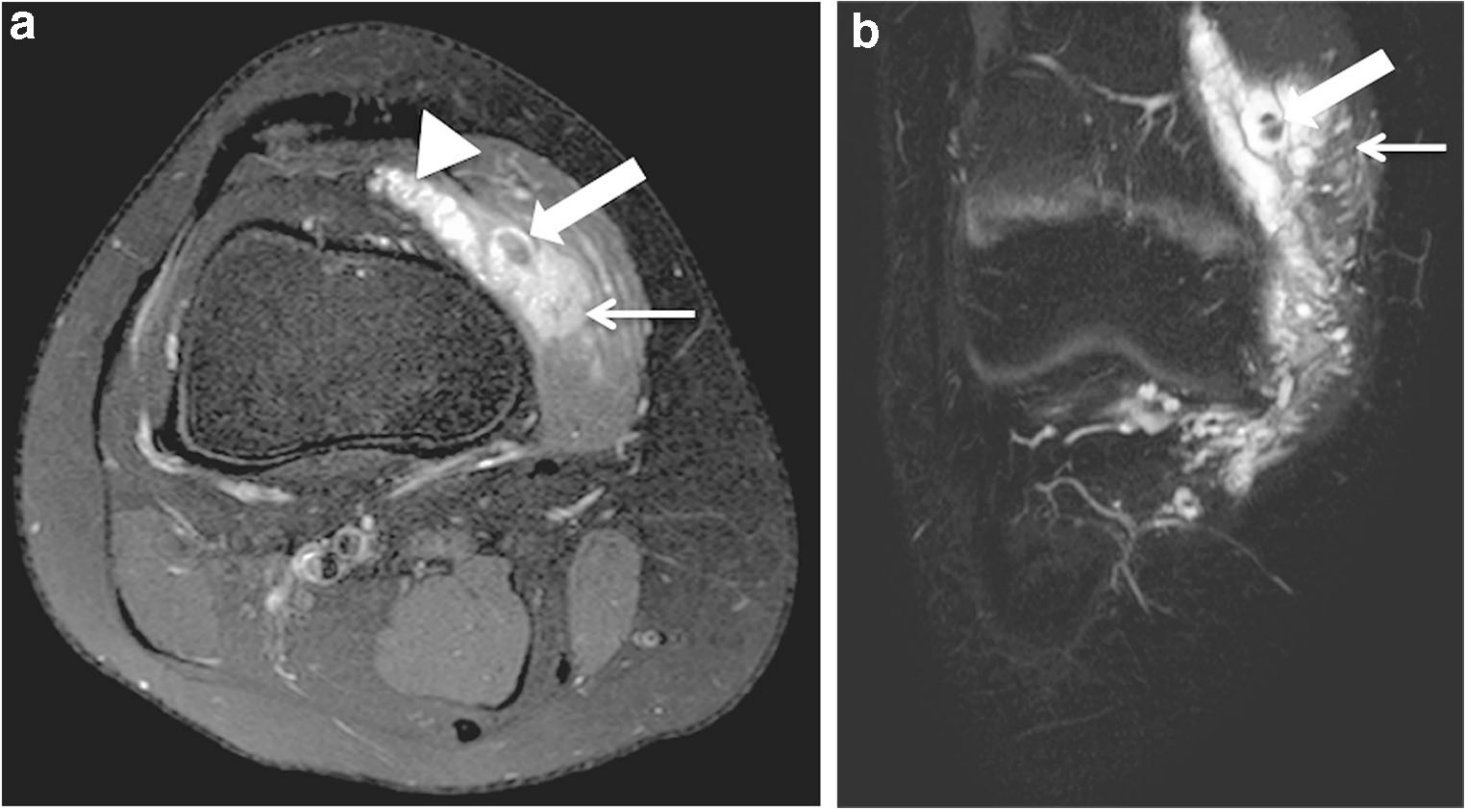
A 13-year-old girl with a venous malformation of the knee that affects both intra- and extracapsular tissues. a, b T2-weighted fat-saturated axial (a) and coronal (b) magnetic resonance images demonstrate the venous malformation invading the distal end of the vastus medialis muscle (*thin arrows*) and the suprapatellar fat pad (*arrowhead*). The small low-signal foci are consistent with intravascular thrombi or phleboliths (*thick arrows*)
